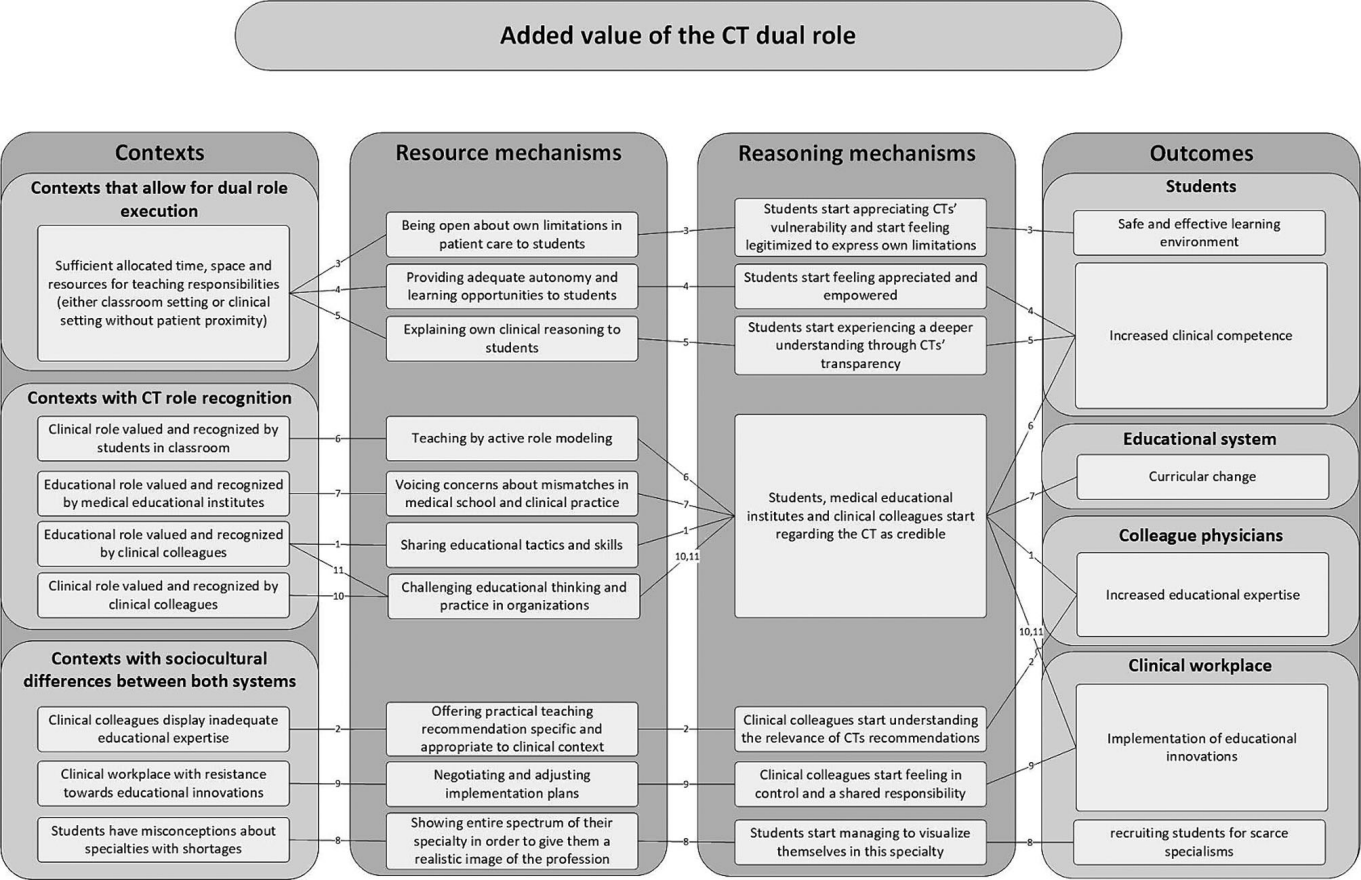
Adjusted programme theory. CT: clinician‐teacher. Numbers in lines correspond with CMO numbers in Table 1.
